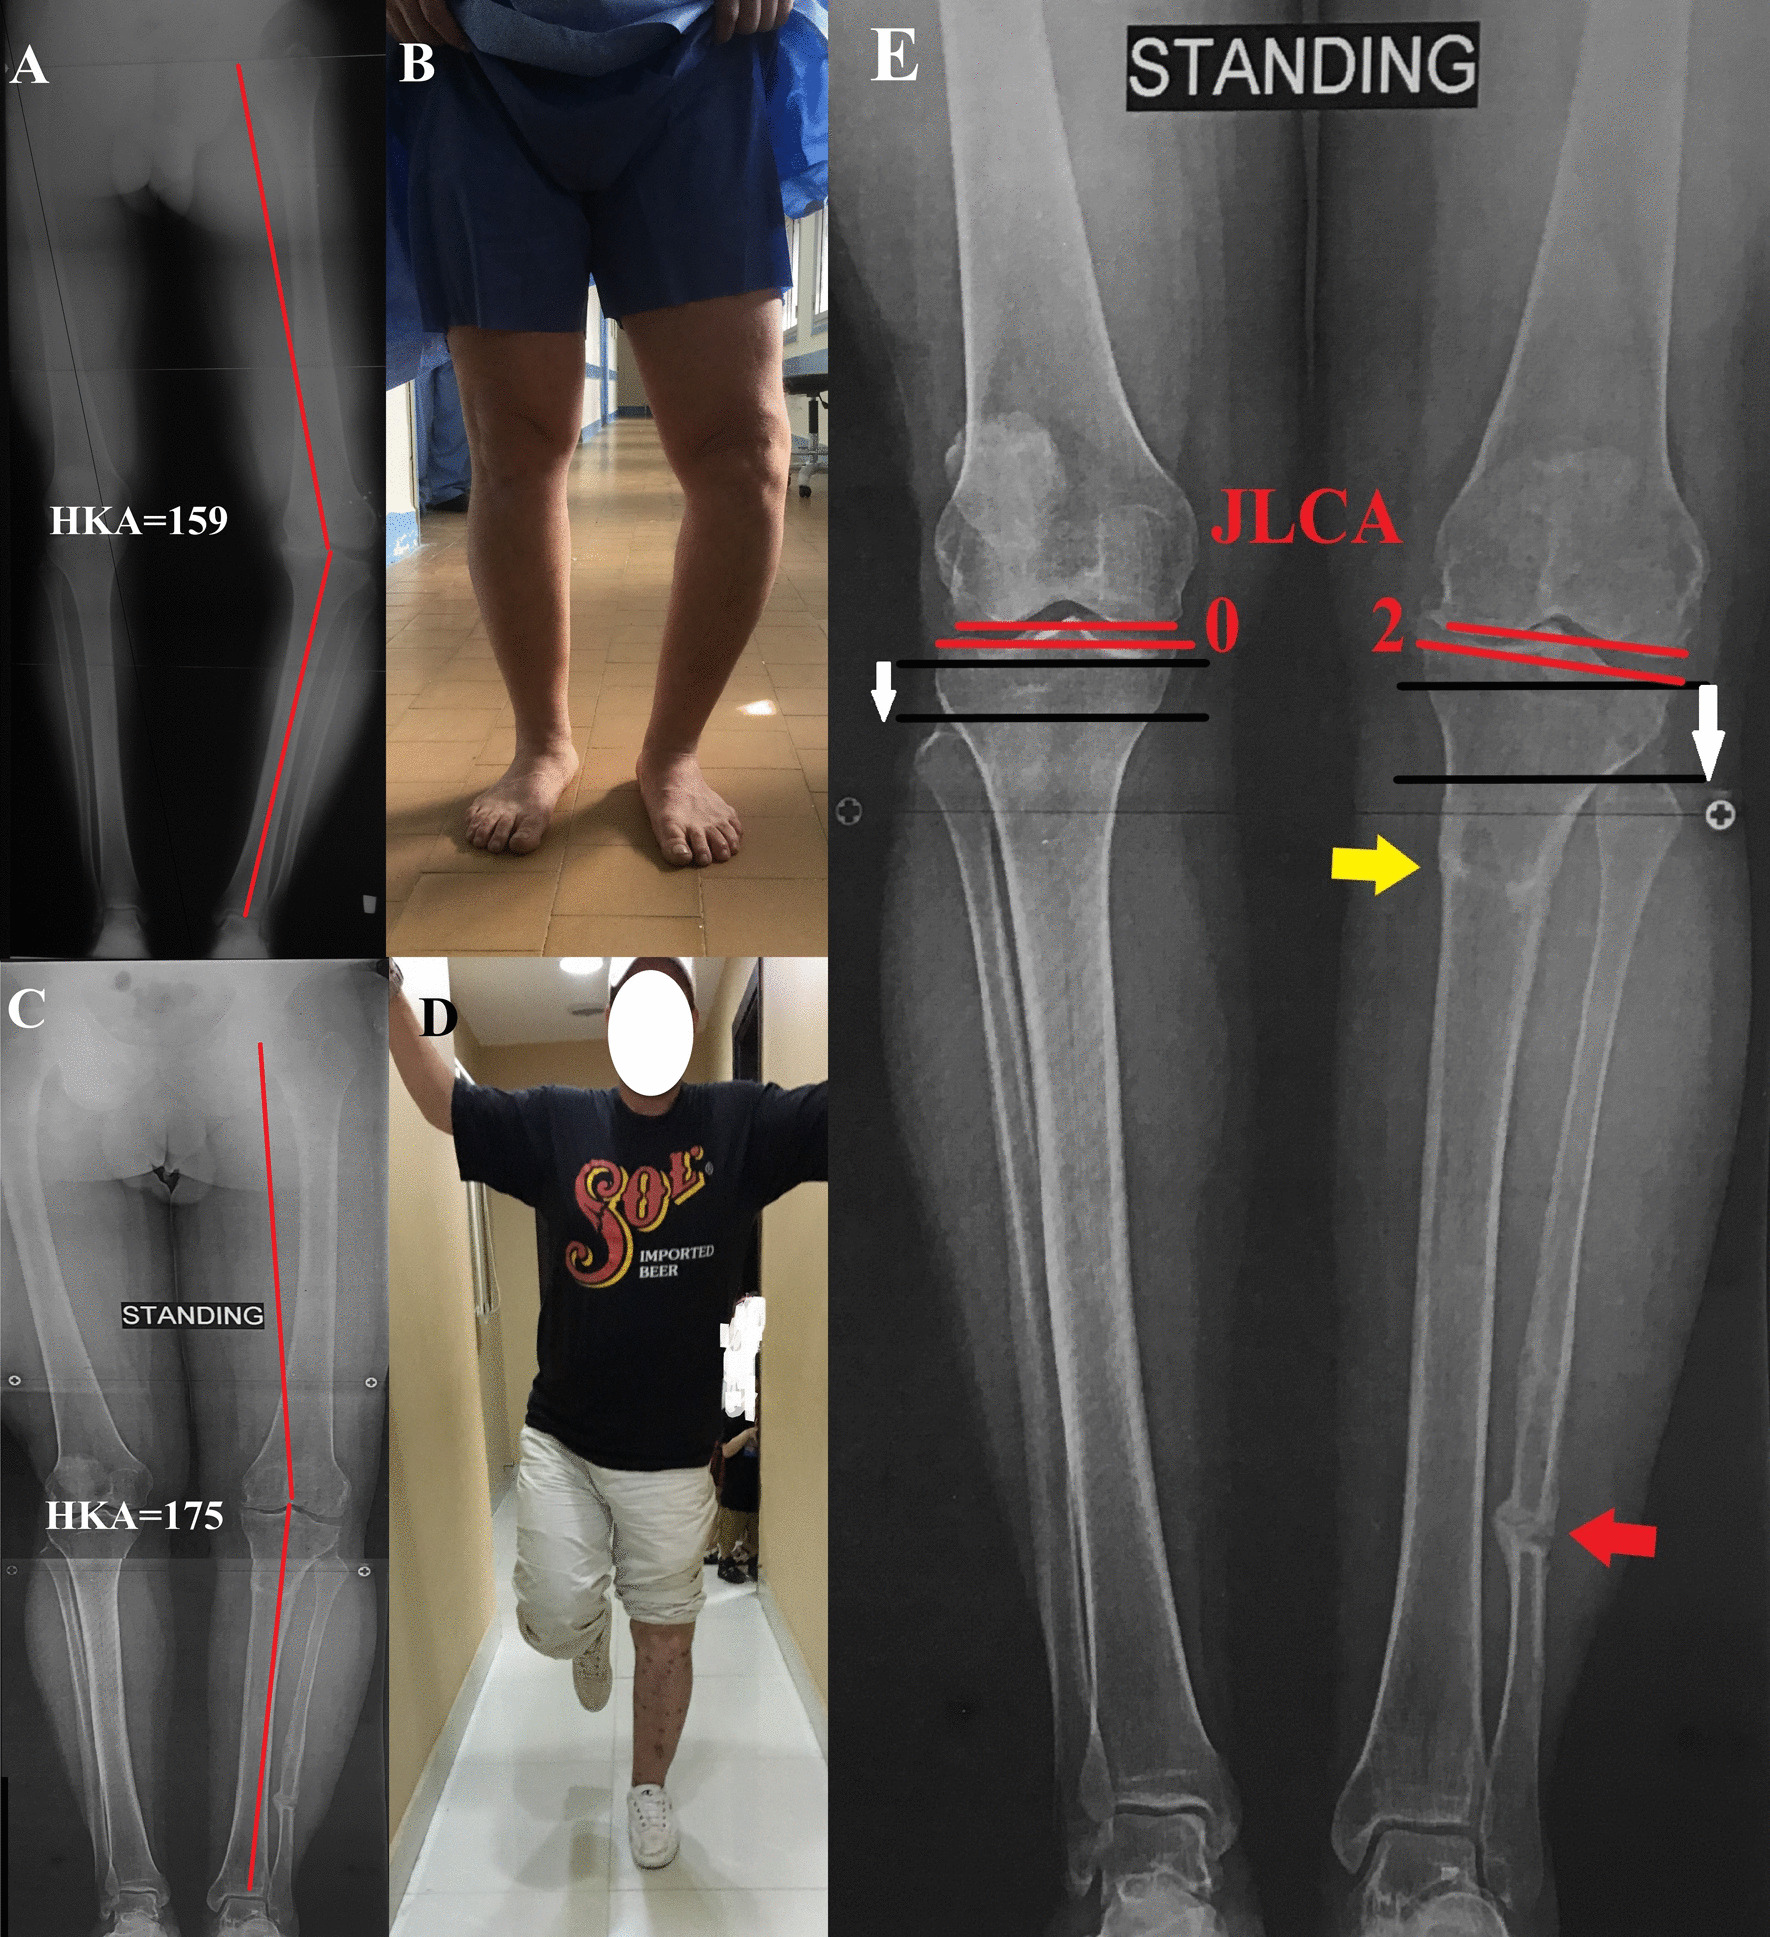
The same patient in Fig. 1. A preoperative radiograph, B clinical image while the patient is standing showing the amount of genu varum deformity. At the final follow-up at 18 months, C long film radiograph showing correction of the deformity to an HKA of 175°, D clinical image while the patient is standing showing deformity correction and indicating knee stability. E comparing the operated knee (left) with the normal side showing near normal JLCA (red lines), healing of the tibial osteotomy (yellow arrow), healing of the fibular osteotomy (red arrow), and the amount of fibular head distalization compared to the normal side (white arrows)
